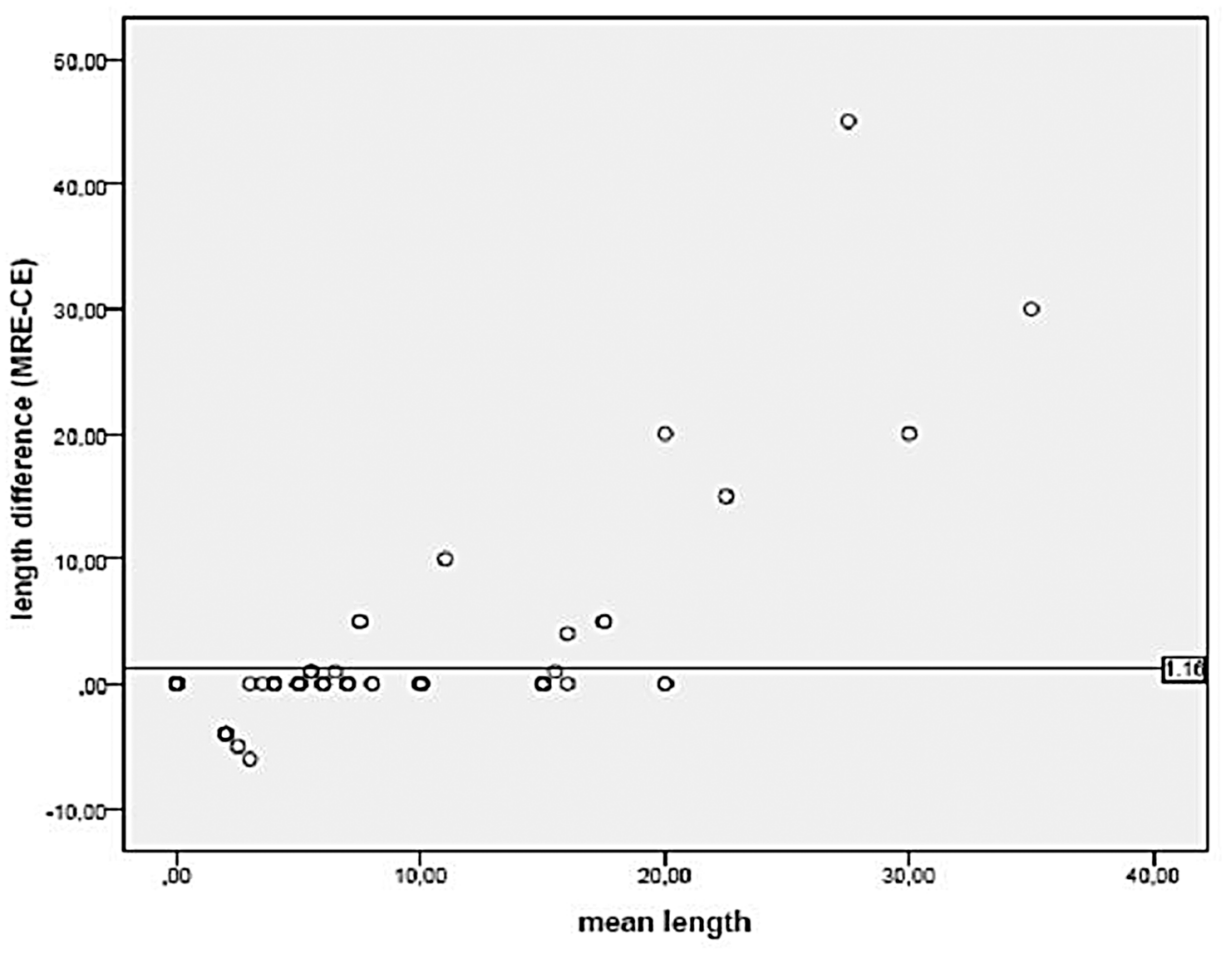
The relation between the mean length and the difference in length of the affected small bowel measured by MR Enterography and Contrast Enhanced Ultrasound.
